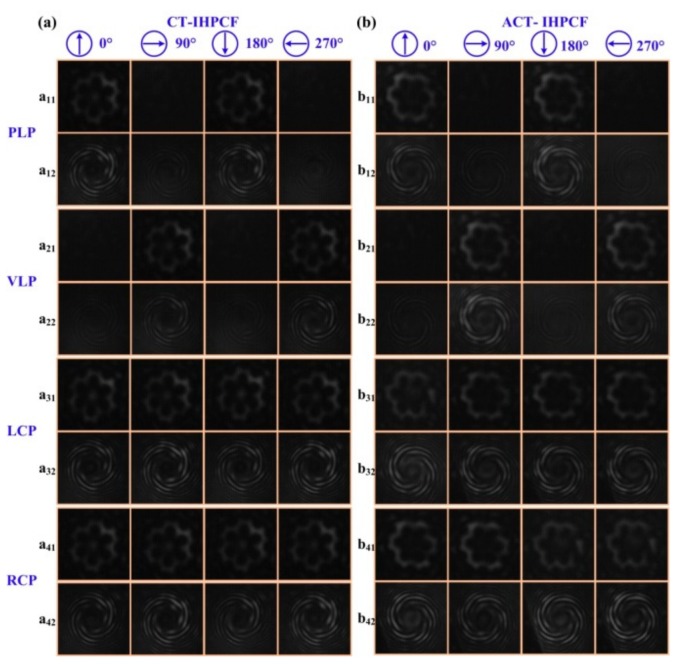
OAM_±6_ modes generated by the (a) CT- and (b) ACT-IHLPFG in the PCF at different polarization states of the input light [15].
